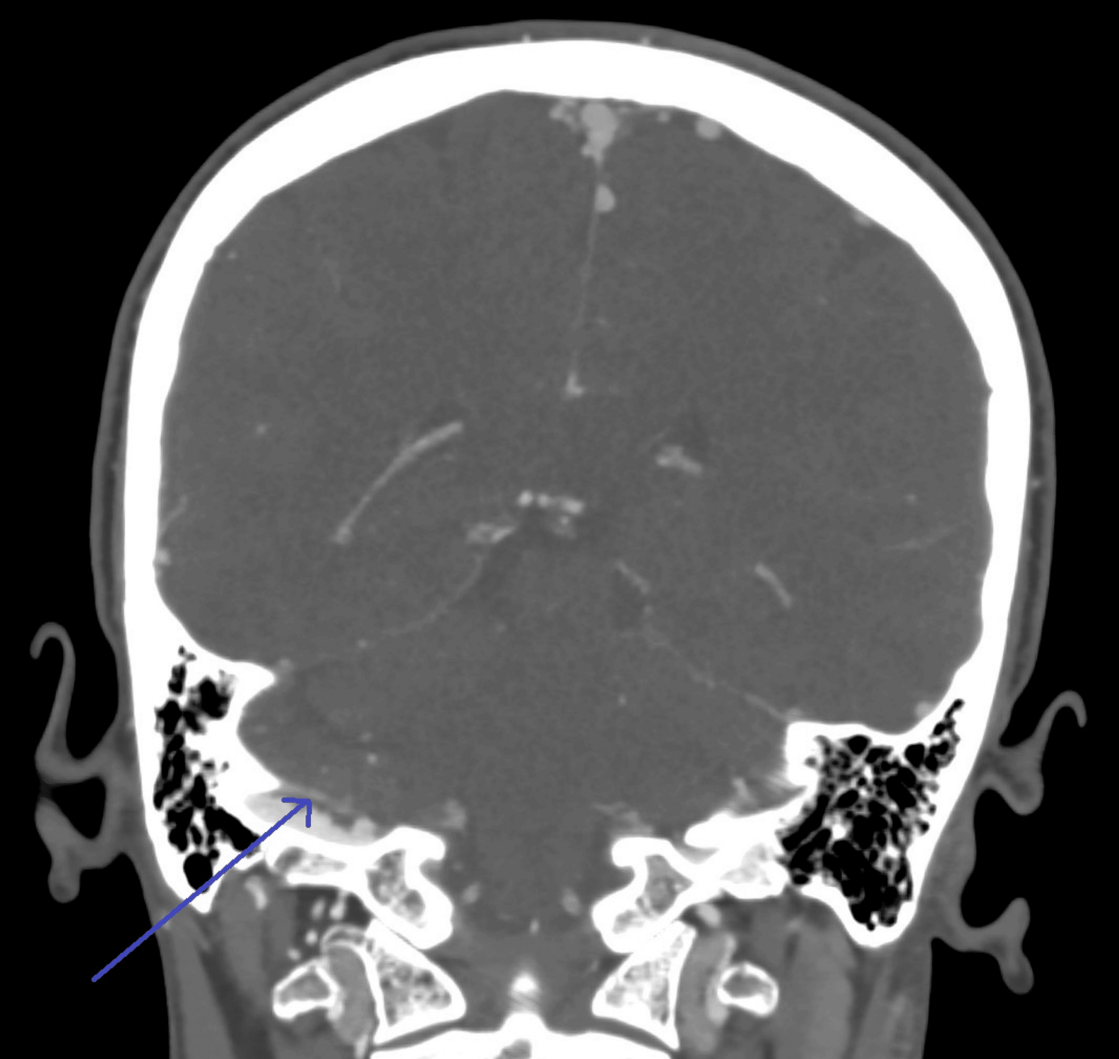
CT venogram showing a linear, eccentric thrombus against the superior wall of the right sigmoid sinus. Thin linear filling defects are present in the bilateral sigmoid sinuses, possibly representing chronic sinus thrombosis. The blue arrow indicates the linear eccentric thrombus.
